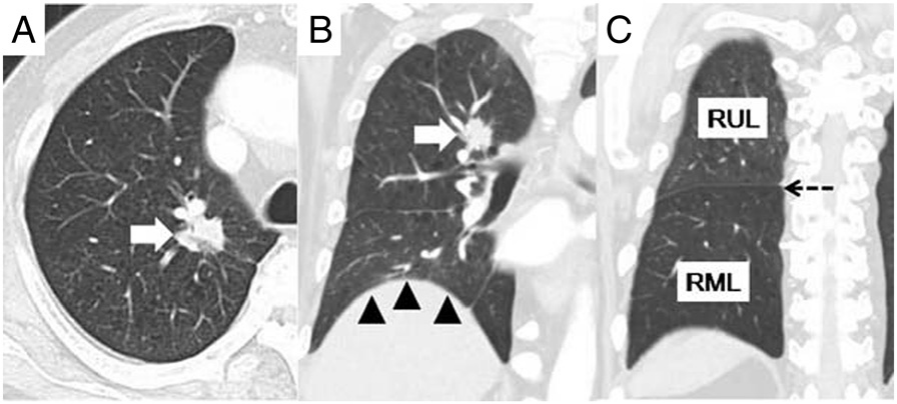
Initial chest computed tomography. (A) Preoperative chest computed tomography (CT) demonstrates a 30-mm mass shadow (arrows) in the right upper lobe, and the distance between the tumor and the entrance of the upper bronchus is 20 mm. (B, C) Compensatory hypertrophy of the middle lobe and elevation of the right diaphragm (arrowheads), reducing the size of the thorax. Dotted arrow revealed interlobar fissure between right upper and middle lobe. RUL, right upper lobe, RML, right middle lobe.
